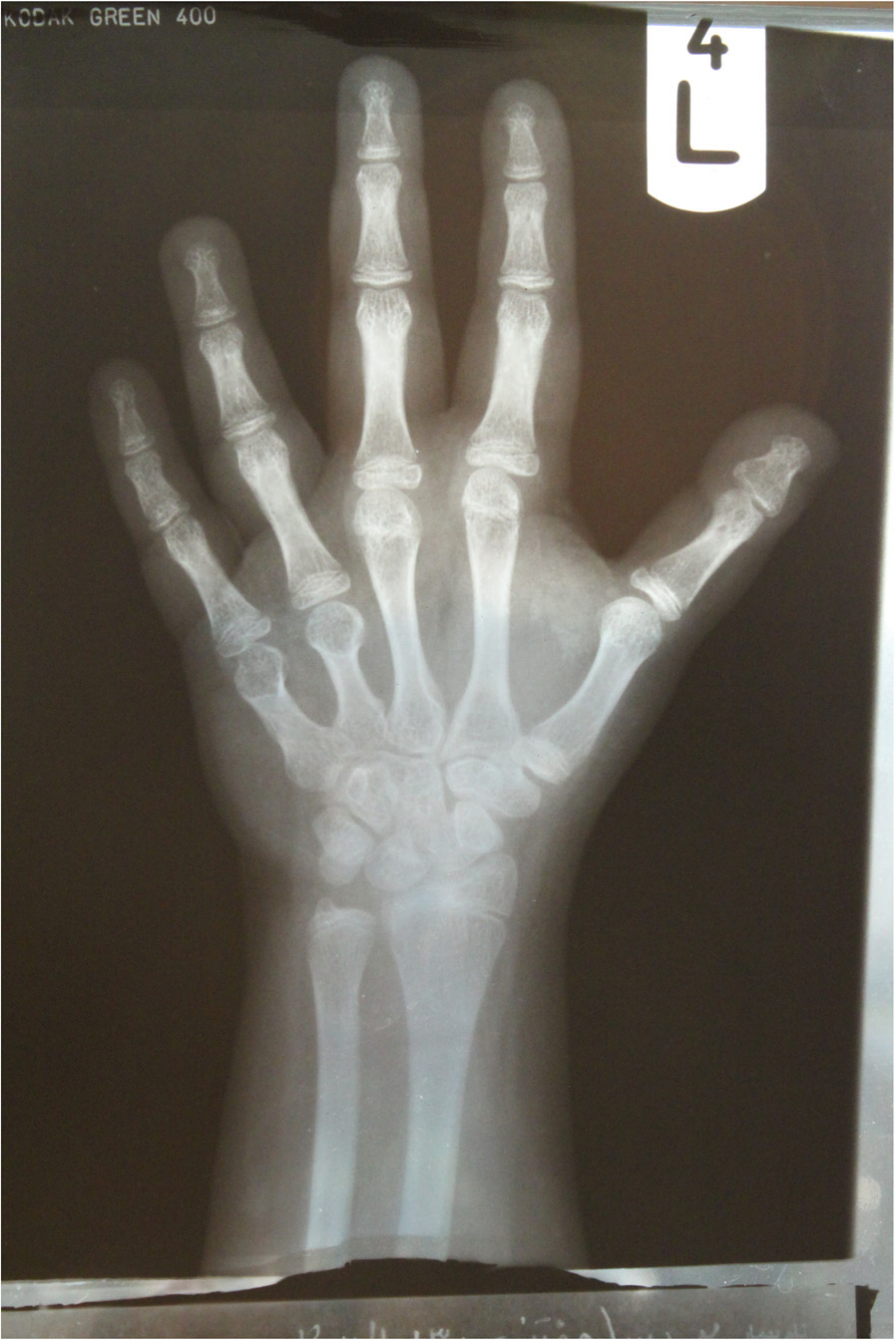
Left hand x-ray of case. Short 4^th^ & 5^th^ metacarpal.
